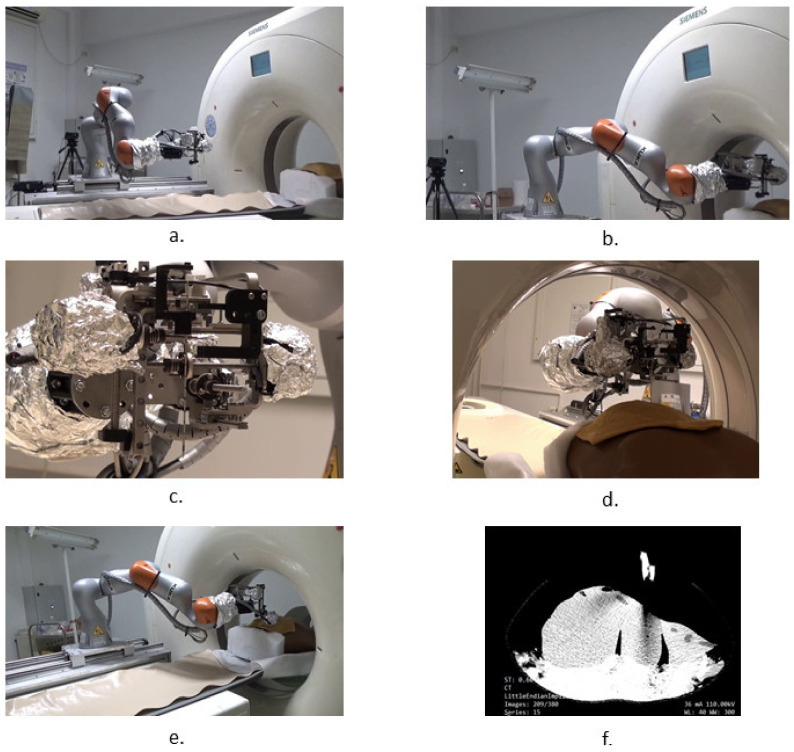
Snapshots during the robotic system validation tests: (a) MNID driven to the insertion site, above the patient; (b) Registration process; (c) Needle gripping; (d) Needle insertion; (e) CT scanning procedure; (f) CT image with the liver and needles.
